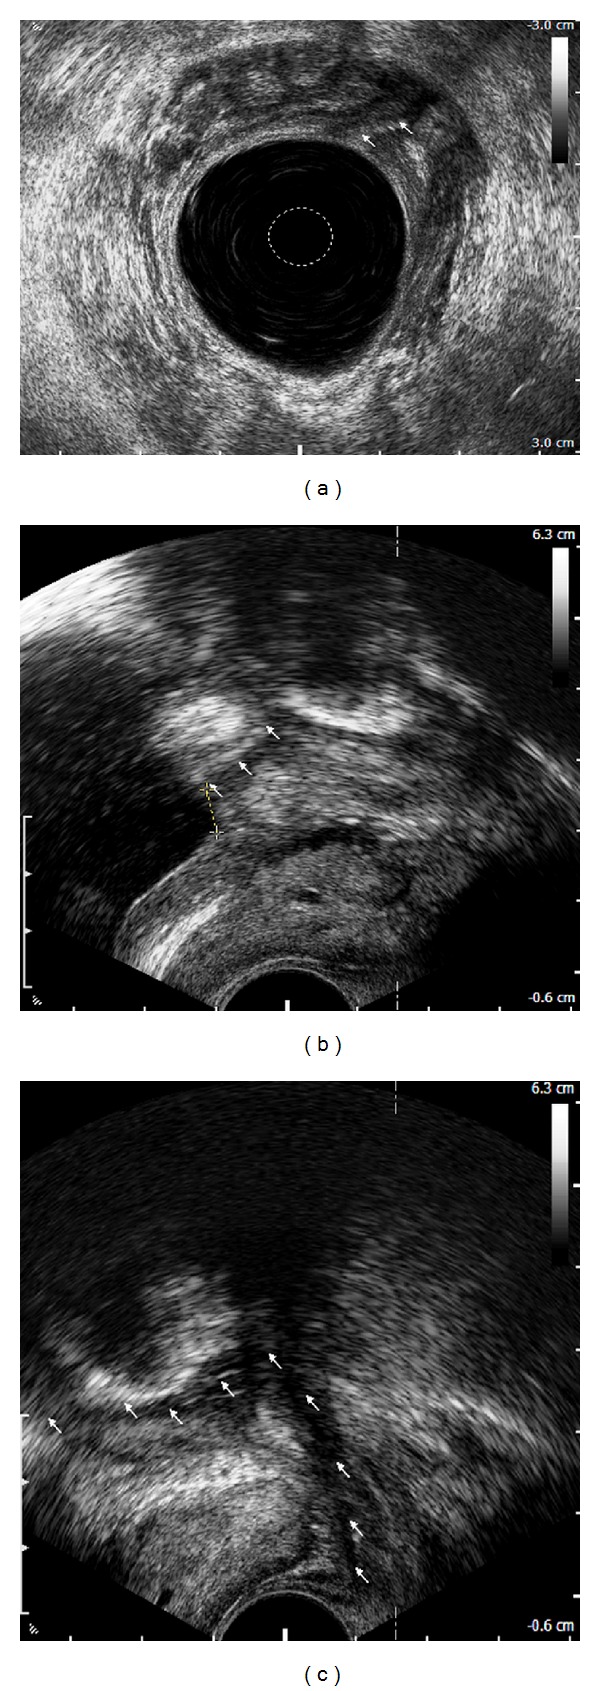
Rectovesical fistula: anorectal endosonographic view of a fistulous orifice in the urinary bladder (arrows) (a), transrectal ultrasonographic view of a fistulous orifice (arrows) located 6 mm from the internal outlet of the bladder (crosses) (b), and transrectal ultrasonographic view of a fistulous tract adjacent to the left lobe of the prostate (arrows) (c).
